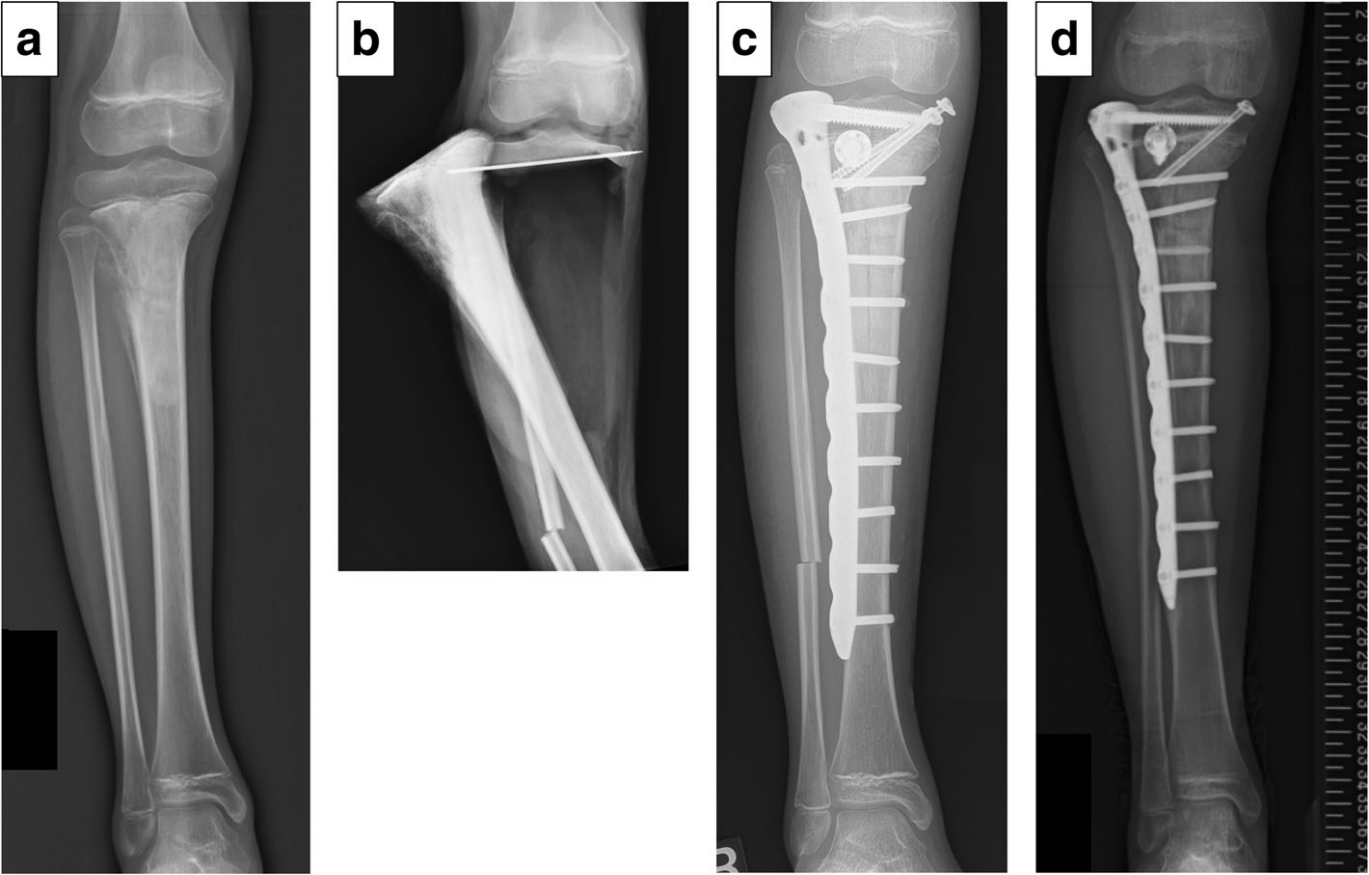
Patient No. 13. A 10-year old girl with osteosarcoma of the right proximal tibia. Preoperative plain radiograph (epiphysis width: 28.3 mm) (a). A radiograph after transepiphyseal excision without osteotomy of the distal tibia for the pedicle freezing. A 1.6-mm Kirschner wire was inserted as a guide, positioned under fluoroscopy. Osteotomy of the distal fibula was performed to expose the proximal part of the tibia (b). A radiograph after the reconstruction using a tumor-bearing frozen autograft stabilized with locking plate (aLDFA: 87°) (c). Whole-leg radiograph after 37 months (aLDFA: 86°, epiphysis width: 33.9 mm) (d)
